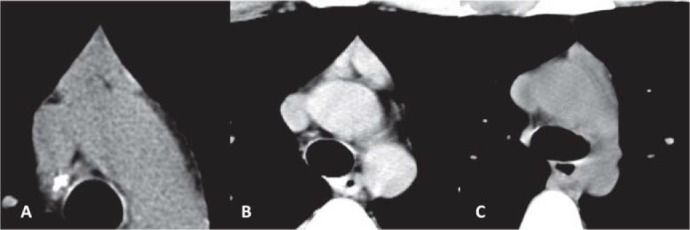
The shape of normal thymus in adults. (A) "Arrowhead" configuration, when the two lobes are confluent. 17-y/o female with myasthenia gravis; CT scan shows remaining thymic mass with density similar to muscle; pathologic diagnosis - thymic hyperplasia. (B) "Bilobed thymus" with two separate lobes. 22-y/o male with myasthenia gravis; CT scan shows diffusely enlarged thymus with higher density than the chest wall musculature; pathologic diagnosis - benign thymoma. (C) One lobe visualized. 31-y/o female with myasthenia gravis; CT scan shows remaining thymic mass; pathologic diagnosis - thymic hyperplasia [4].
